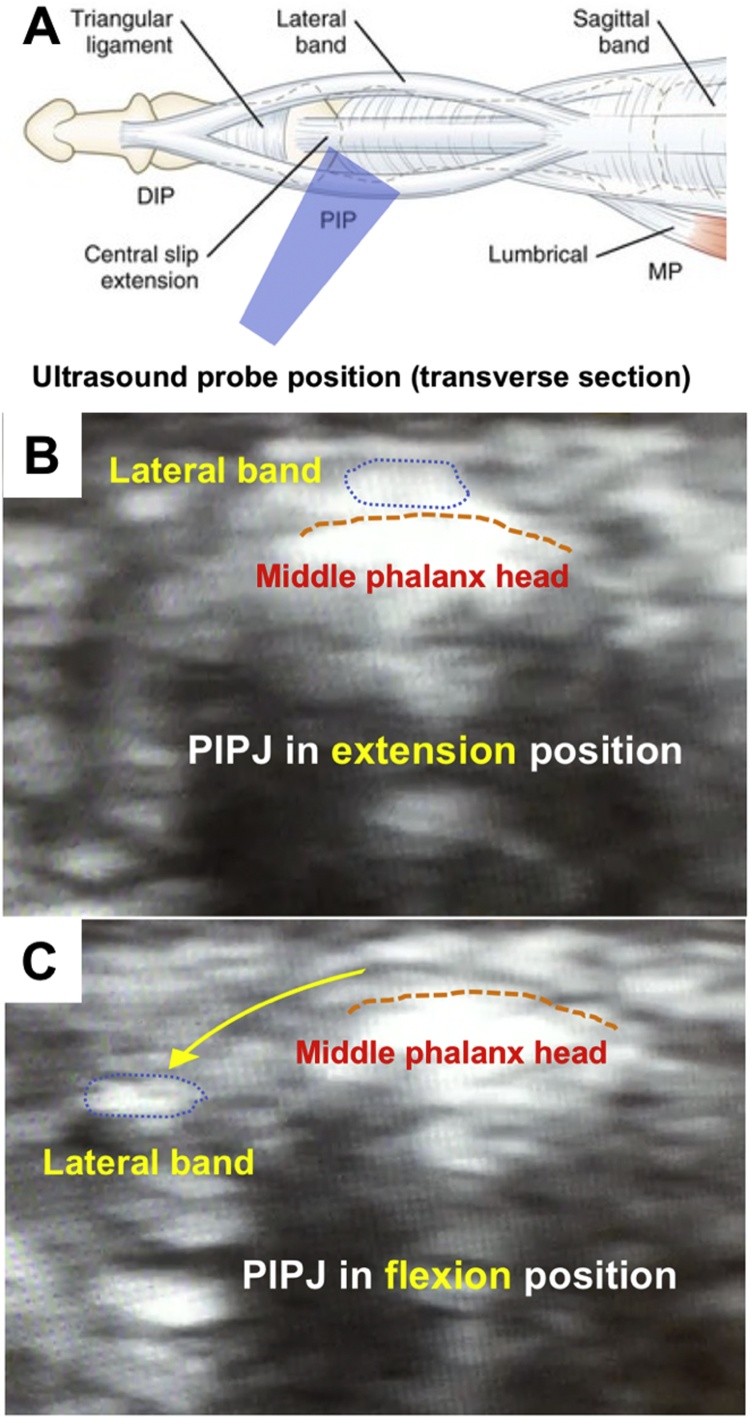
Dynamic change of lateral band under ultrasonography (A: Probe placement over dorsal and radial side of middle phalanx head as transverse section; B: Lateral band in situ over middle phalanx head upon extension of PIPJ; C: Subluxation of lateral band to radial side upon flexion of PIPJ.
